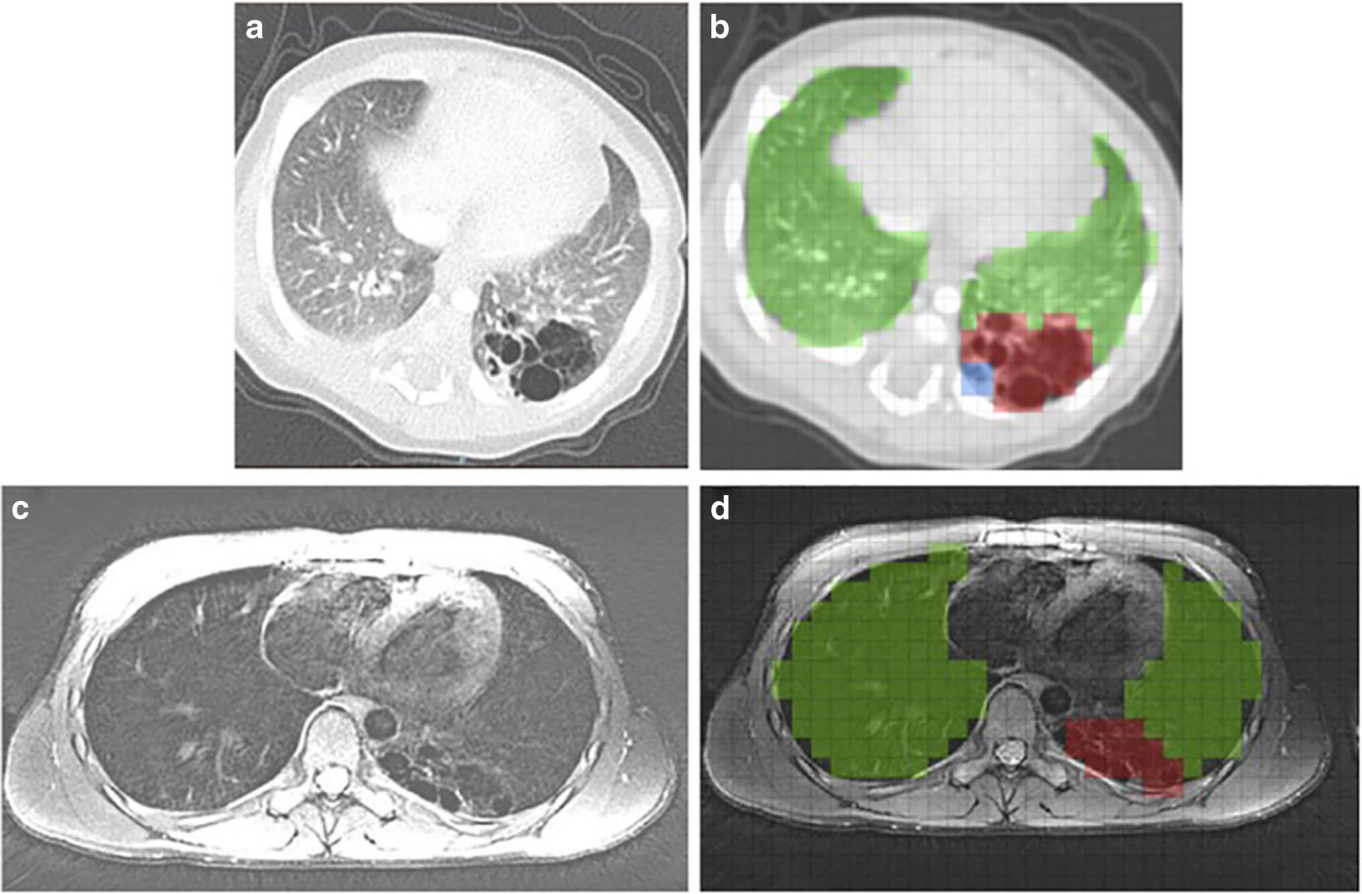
Example of an axial postnatal CT (a) and school-age MRI (c) in a patient with a CPAM of the left lower lobe. Both images show a multicystic, air-filled lesion. CLAQ scoring (b) shows normal lung tissue (green), lesion-associated parenchymal abnormalities (atelectasis, blue) and the lesion (red); the CLAM scoring (d) shows normal lung tissue (green) and the lesion (red)
